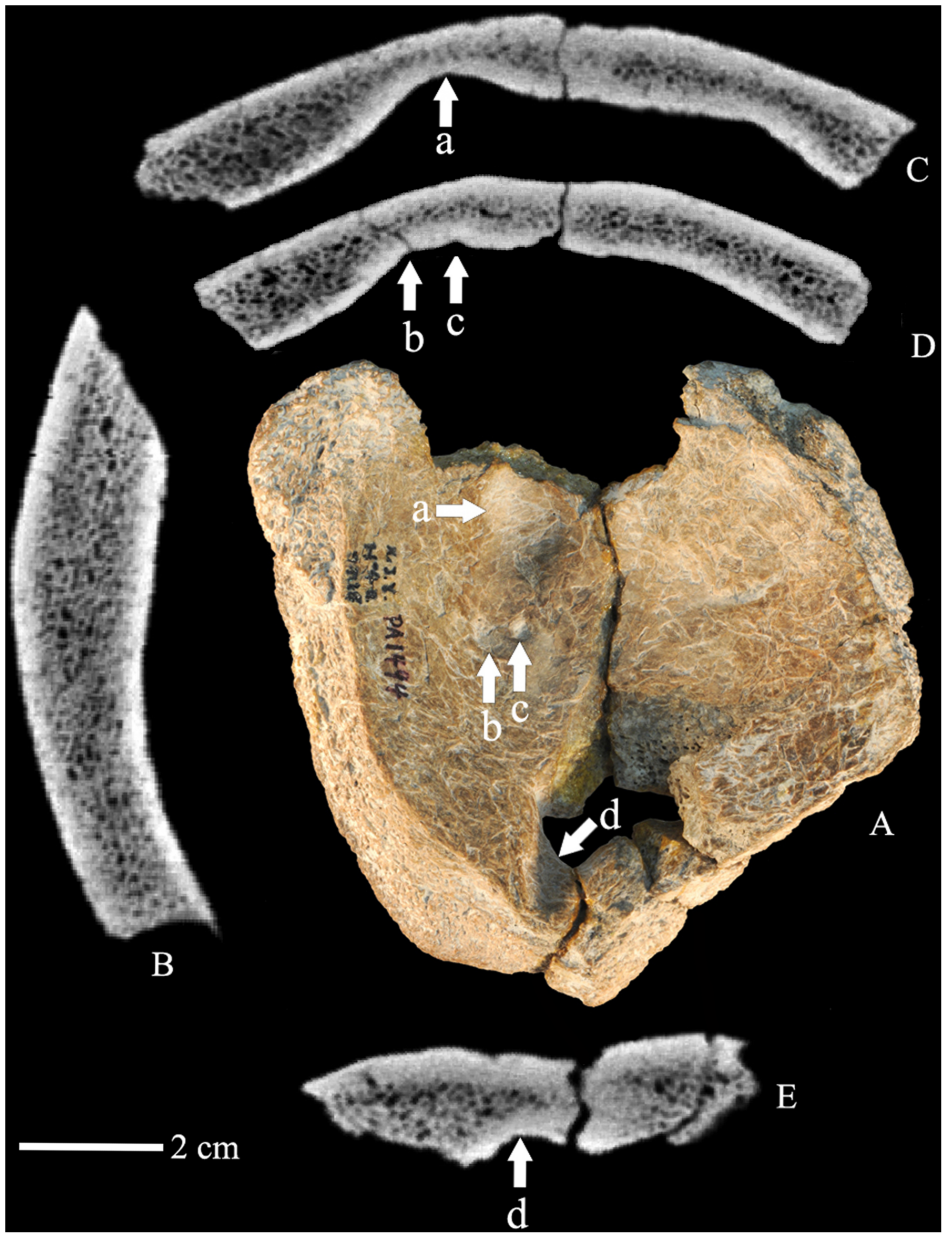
The Xujiayao 11 parietal bones. Interior view (A). CT sagittal section image showing the thickness of the bone (B). CT coronal section images (C, D, E) showing the large Pacchionion depression (a), the two small granular foveolae (b, c) and the wide venous sulcus (d) in the bone. Anterior is above.
